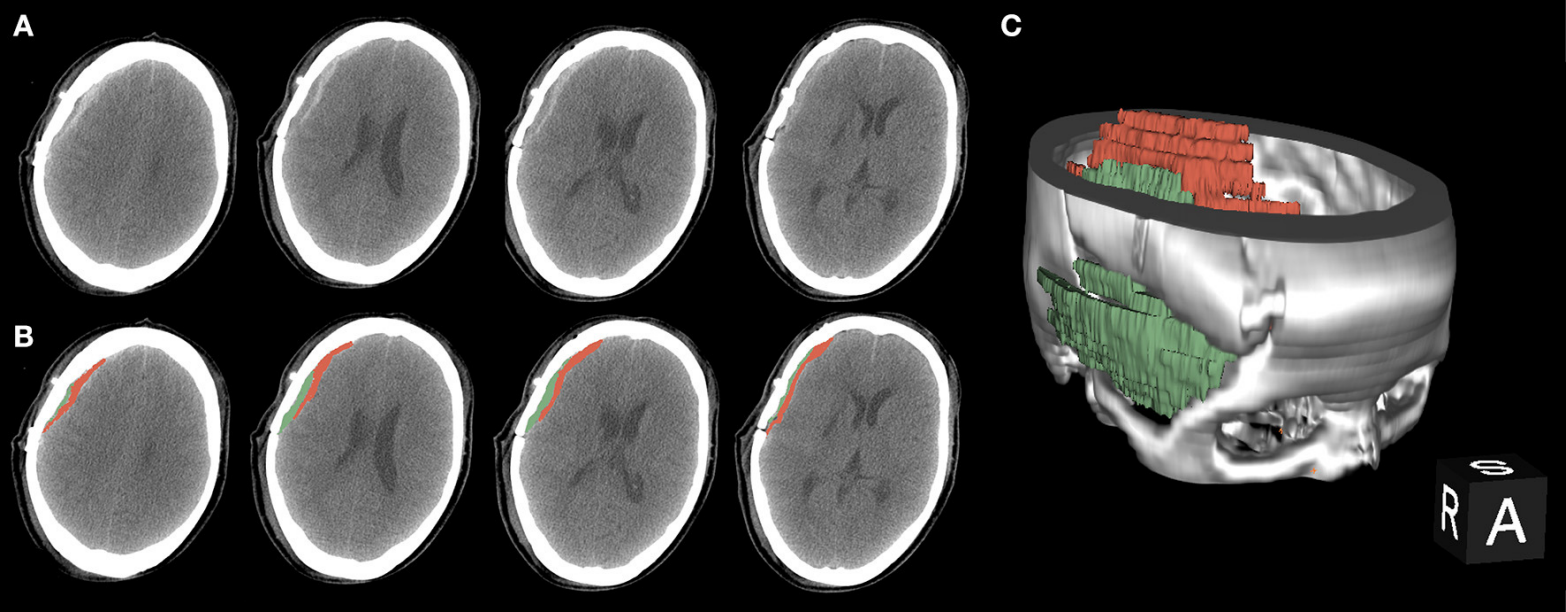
A procedure for typical bloody fluids around the operative area. (A) Original presentation of bloody fluids on post-operative computed tomography (CT) examinations. Black arrows indicate the separated temporal muscle. The graph shows different CT values with adjacent bloody fluids. (B) Bloody fluids are marked in red and the temporal muscle is marked in green using the 3D Slicer software. (C) The location of bloody fluids and the temporal muscle *via* 3D rebuilding.
